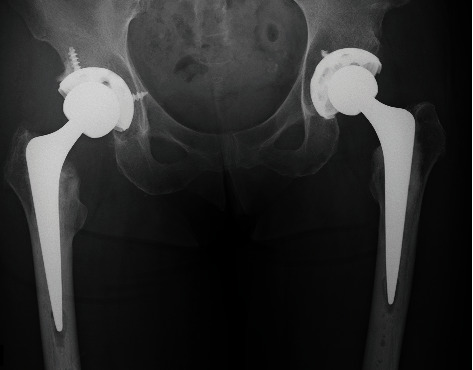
Postoperative plain radiograph. Hybrid THA was performed on both hips.
